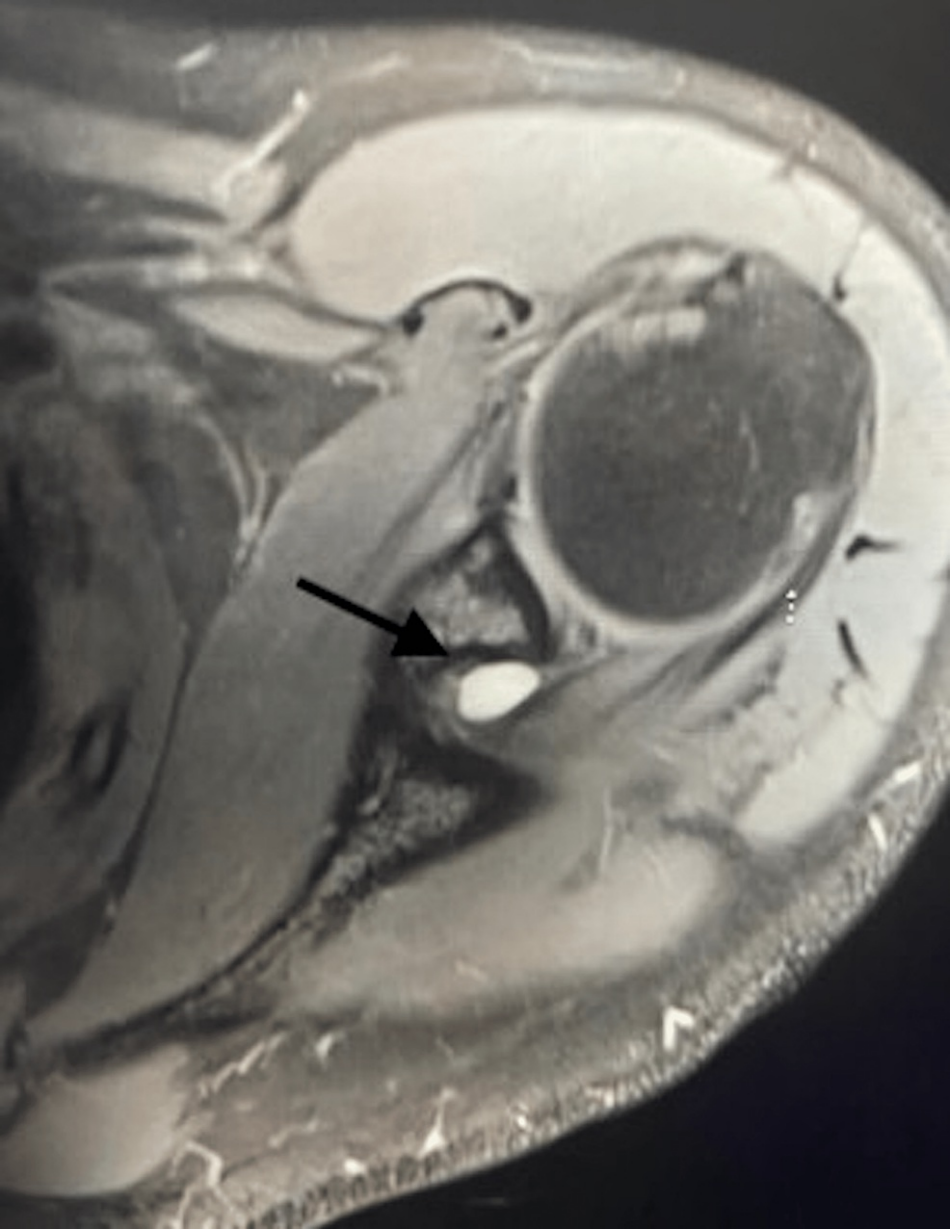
Axial MRI scan of the left shoulder showing the spinoglenoid notch ganglion cyst
